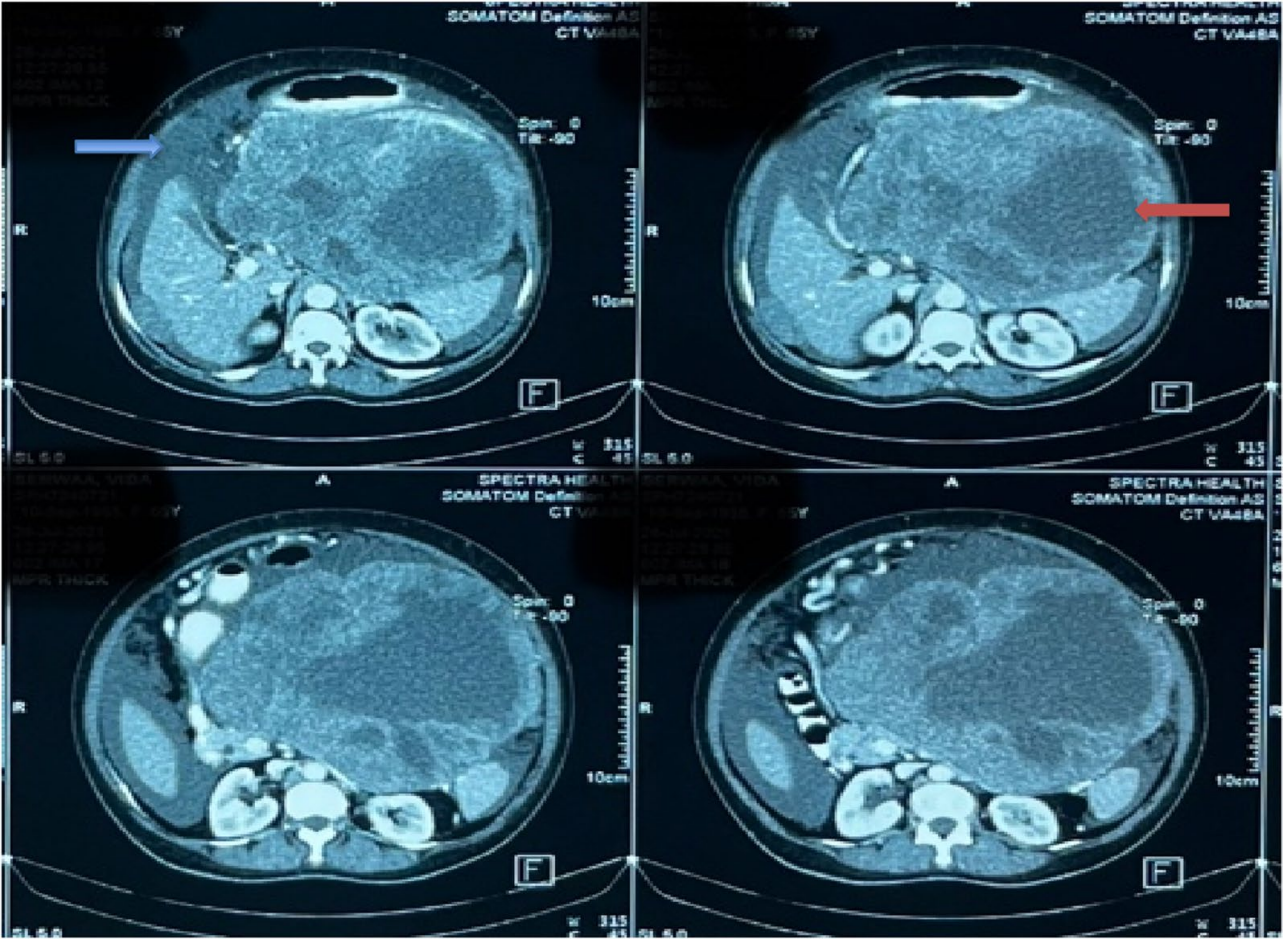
Abdominal CT scan showing heterogenous gastric tumour (red arrow) with ascites (blue arrow)
